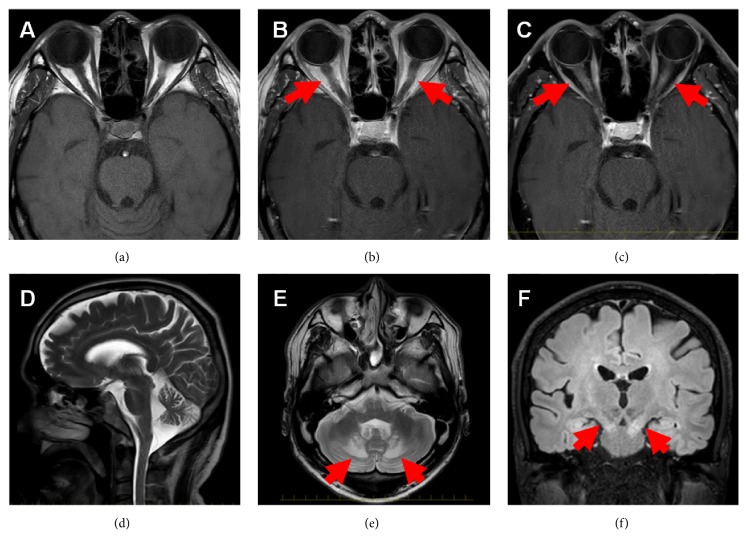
Brain magnetic resonance imaging revealed the following: bilateral swelling of optic nerves on T1-weighted images (a) with enhancement (arrows) on gadolinium-enhanced T1-weighted (b) and T1 weighted fat-suppressed images (c), enlarged fourth ventricle and cerebellar atrophy (d), and hyperintensities in the bilateral dentate nuclei (arrows, (e)) on T2-weighted images; FLAIR images demonstrated hyperintensities in the bilateral dentate nuclei and pyramidal tract (arrows, (f)).
